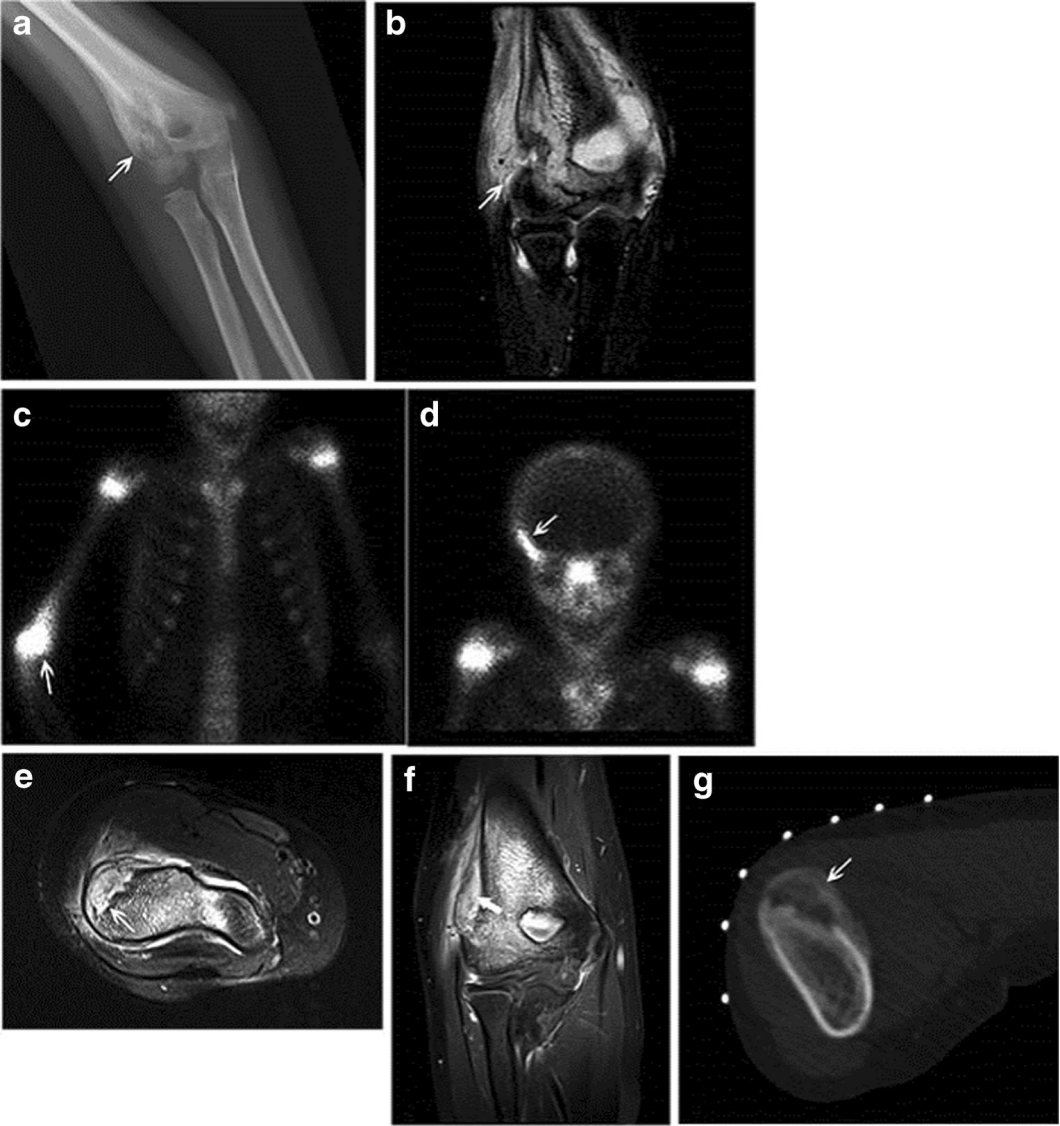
Chronic recurrent multifocal osteomyelitis (CRMO) in an 8-year-old girl. An anteroposterior radiograph of the right elbow a reveals an eccentric, mostly lucent lesion at the lateral distal humerus (*arrow*) with surrounding periosteal reaction. Corresponding coronal T2 STIR image b reveals hyperintense, abnormal signal eccentrically along the distal lateral humerus with overlying periosteal reaction and surrounding soft-tissue edema. Tc-99m-labeled MDP bone scan reveals increased radiotracer uptake at the distal right humerus c and the right sphenoid d, suggesting multifocality of the process. The patient had a chronic course with recurrent exacerbations. Axial STIR e and coronal T1 post-contrast f MR images obtained 2 years after the initial presentation reveal STIR hyperintense, enhancing abnormality along the lateral distal humerus (*thin arrows* on e, *thick arrow* on f). The abnormality is peripheral, and involves the cortex and overlying periosteum. A non-contrast axial CT image g obtained at the same time for biopsy guidance shows cortical irregularity through the affected portion of the lateral distal humerus with significant overlying periosteal reaction (*arrow*)On plain radiographs, lesions are characteristically juxtaphyseal, osteolytic, sclerotic or mixed and lack periosteal reaction [22]. Less commonly, some patients, particularly those with unifocal lesions, show breached cortices and periosteal appositions, in which case distinction from the tumour becomes important [24]. Rarely, diaphyseal lesions with only cortical thickening have been reported [25]. Such periosteal/isolated cortical involvement, though overall uncommon in the setting of CRMO, falls under the category of lesions involving the outer cortical surface of the bone, which are being addressed in this review.Multiple lesions, if not visible radiographically can be detected using radionuclide bone scanning or whole-body MRI — the latter being the more sensitive method of evaluation [25]. Lesions are typically hypointense on T1 and hyperintense on T2 [25]; MR is also useful for detection of synovial enhancement near the bone lesions [26].
